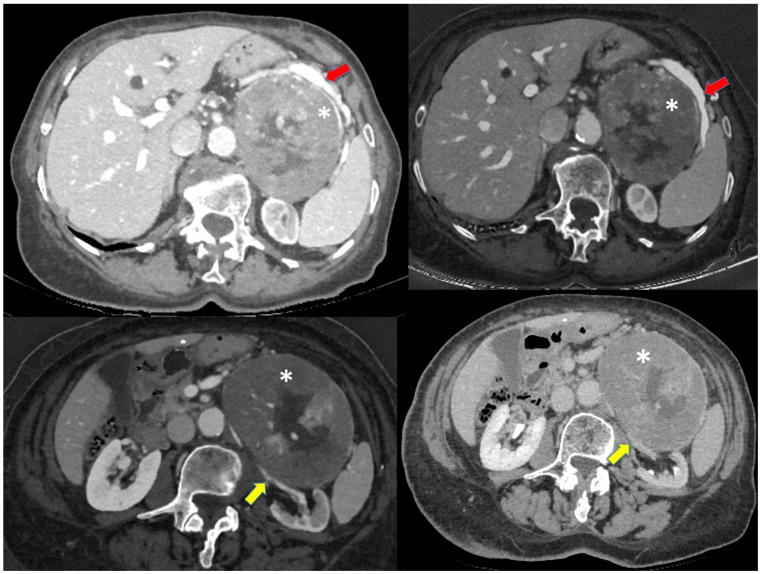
CT-scan image: left adrenal mass capsulated and not homogeneous, displacing the pancreatic body and the splenic vessels anteriorly and the kidney and renal vessels inferiorly. The red arrow indicates the splenic artery displaced by the mass, the yellow arrow indicates the left renal vessels, the asterisk indicates the adrenal mass.
